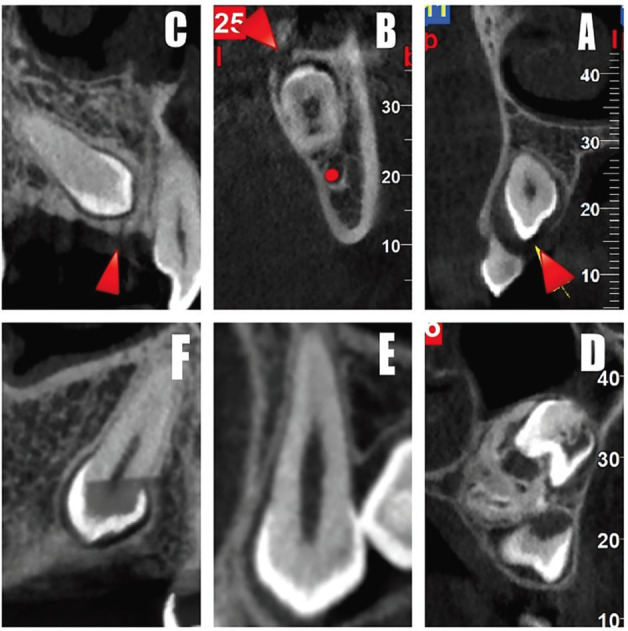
Cone-beam computed tomography images of the gubernacular canal were detected in teeth with normal eruption (A), delayed eruption (B) and impacted tooth (C), and not detected in normal eruption (D), delayed eruption (E) and impacted tooth (F).
